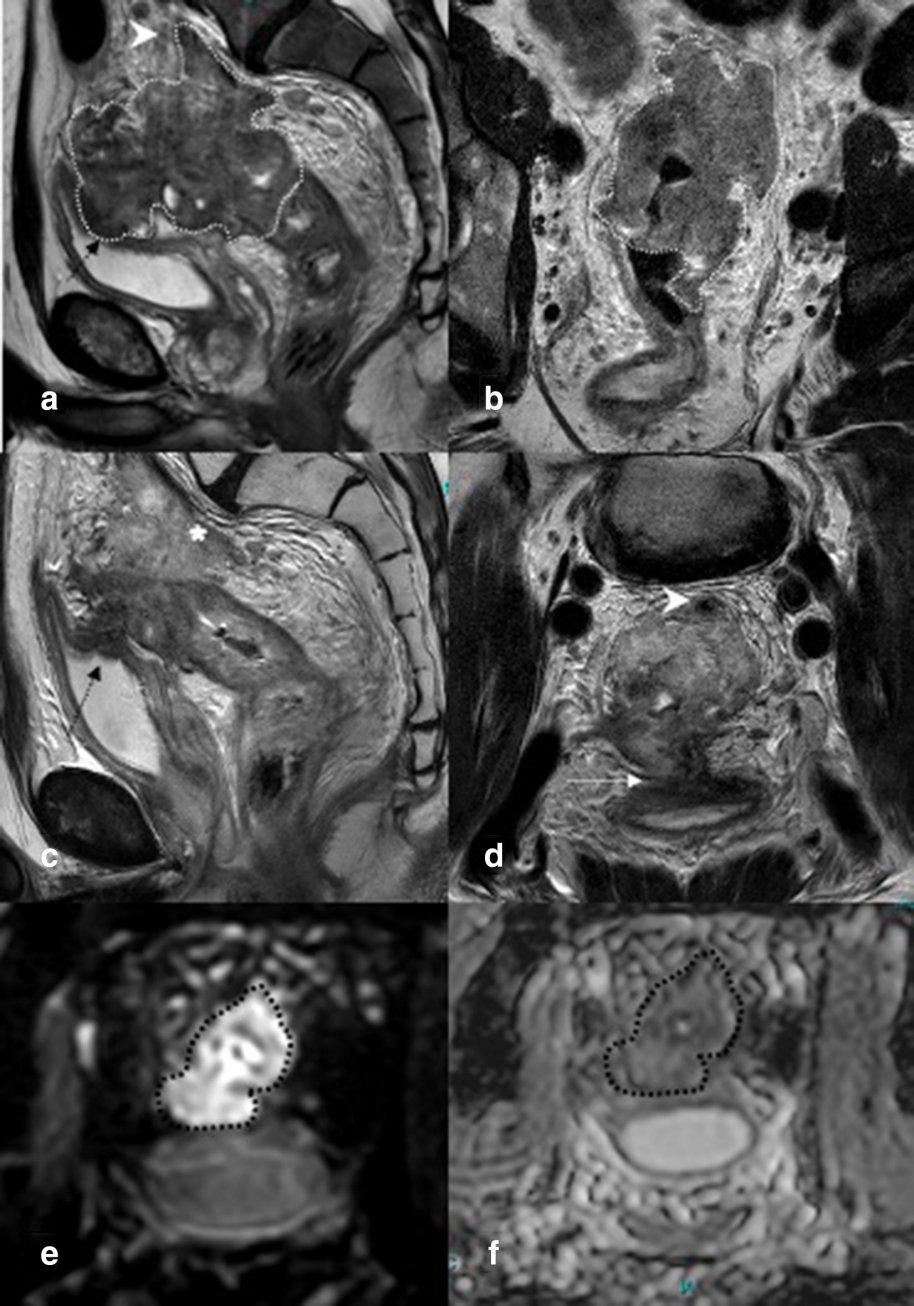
In (a and b), the primary tumor is delineated with a dotted line: a proximal rectal cancer massive extramural growth with EMVI (arrowhead in a) and invasion of the bladder (black arrow in a). After CRT on T2-weighted images (c, d), there is a decrease in volume, but obvious residual tumor is visible, still invading the bladder (arrow in d) and pre-sacral vessels (persistent EMVI, asterisk in c and arrowhead in d). The diffusion-weighted images (e/f) show obvious residual restriction (outlined with a black dotted line), confirming the residual tumor. Endoscopy is not necessary in these cases. EMVI, extramural venous invasion.
